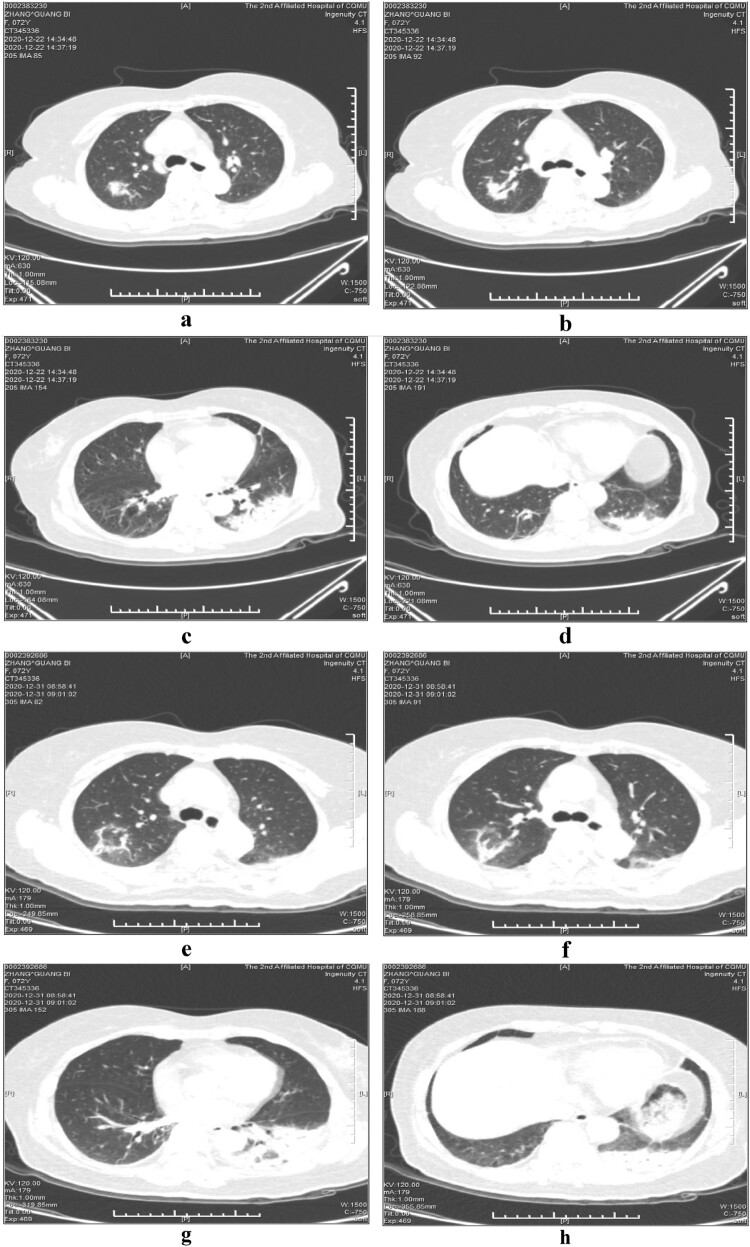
Serial chest computed tomography (CT) scans of the 72-year-old female with psittacosis pneumonia (the first patient). The initial CT scan (4 days after the onset) shows consolidations with bronchograms in the right upper lobe (a, b) and left lower lobe (c, d). The follow-up CT scan (13 days after the onset) shows the area of consolidation in the right upper lobe has decreased (e, f), while the consolidations in the left lower lobe only changed a little (g, h).
